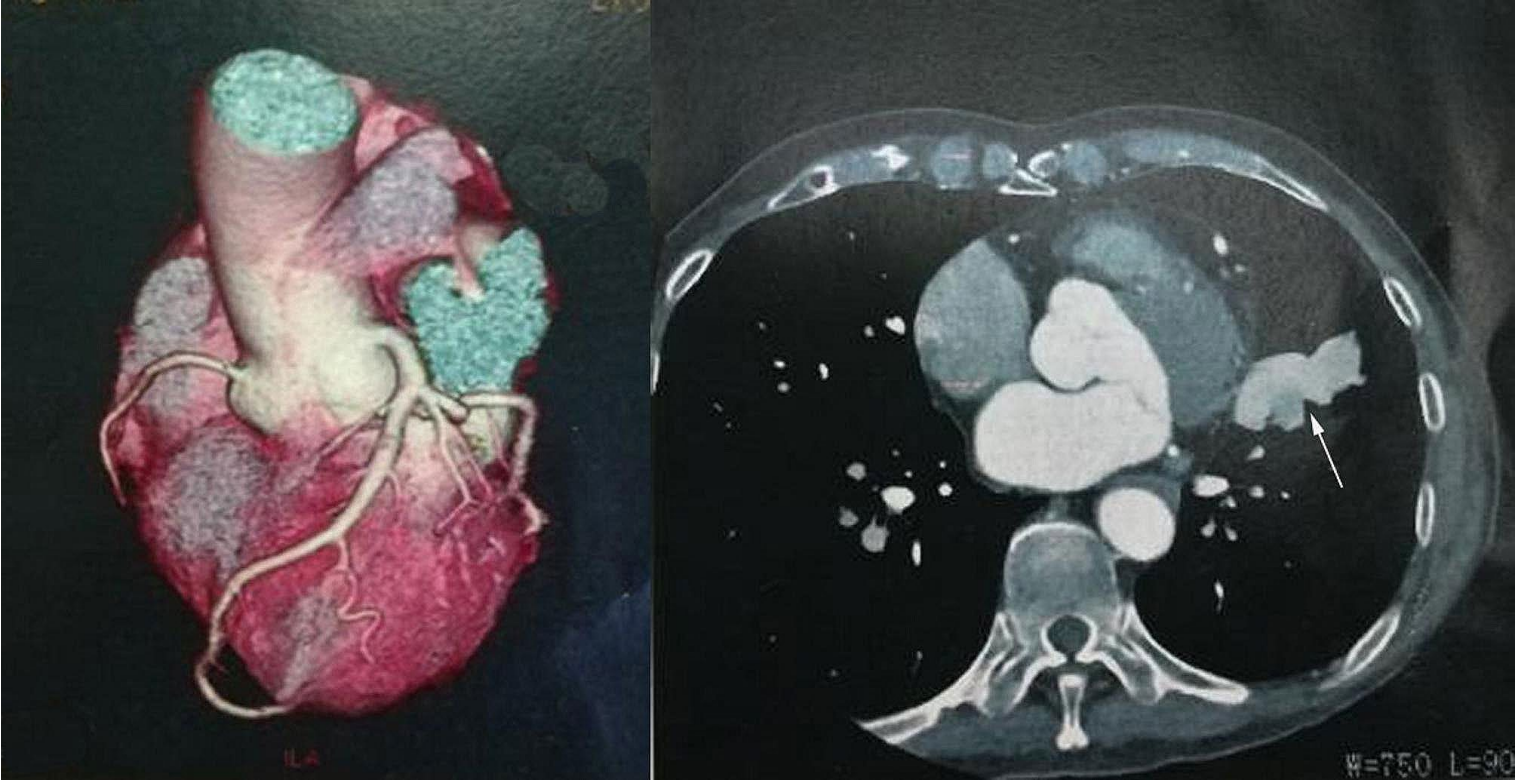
Coronary CTA showing abnormal density shadow of the left upper lobe tongue segment
